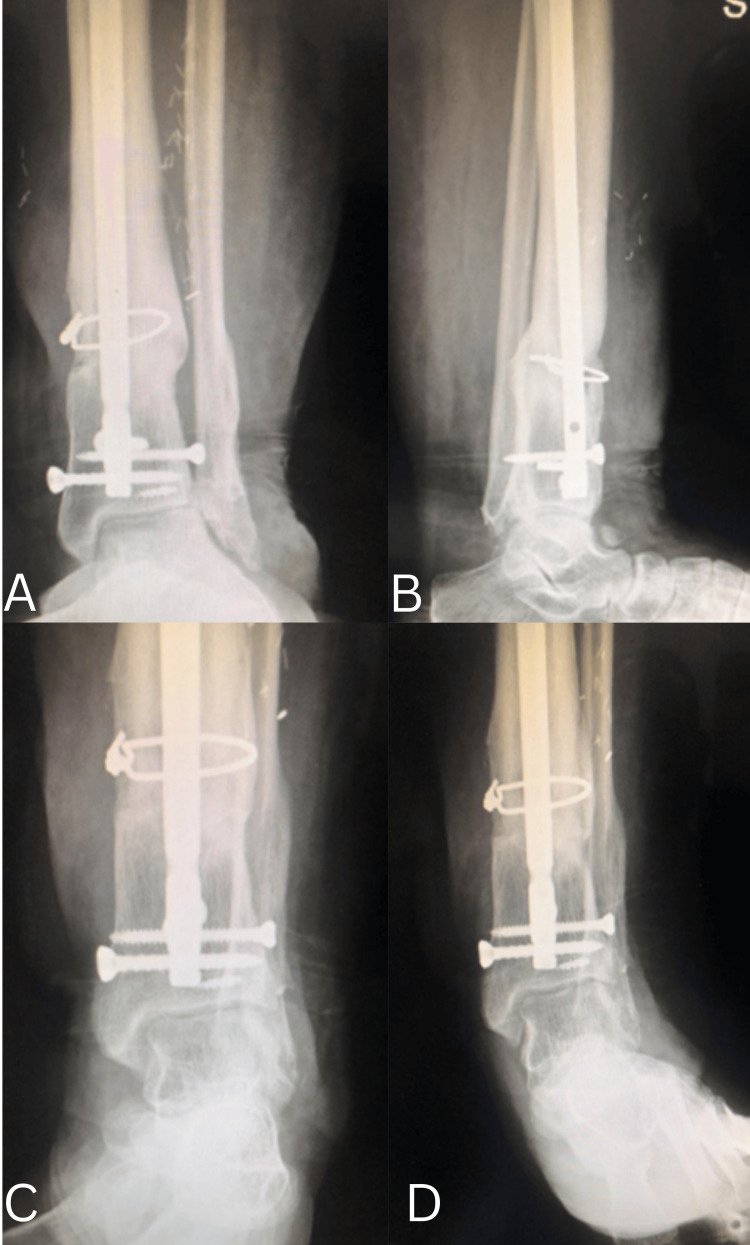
Two-year follow-up radiographs of the distal tibia and ankle A. Anteroposterior view demonstrating complete fracture union with mature callus formation and maintained alignment. B. Lateral view confirming solid consolidation of the fracture with preserved sagittal alignment. C. Varus stress view showing a stable ankle mortise without evidence of instability. D. Valgus stress view demonstrating maintained joint congruency with no medial or lateral talar tilt.
